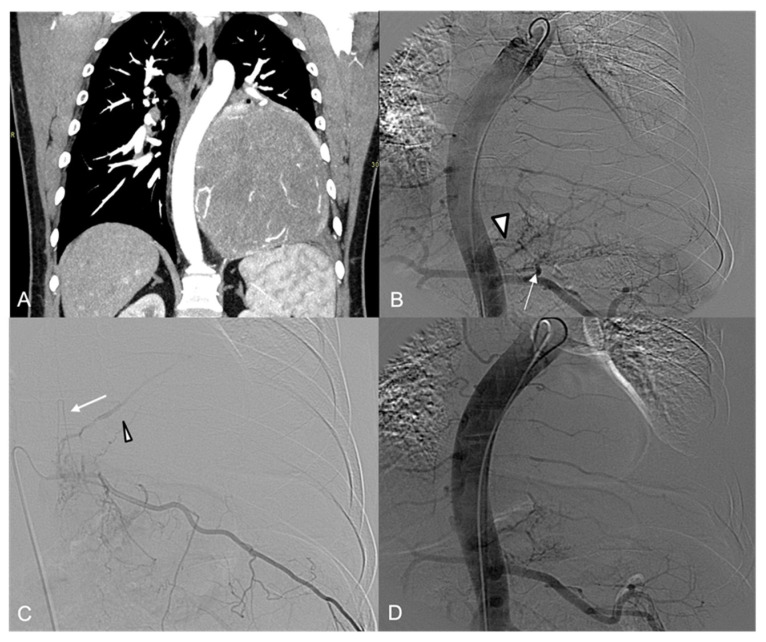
(A) CT Angiography, coronal view: large, highly vascular mass in the left hemithorax dislodging the thoracic aorta. (B) Preliminary aortography shows the rich vascularization of the mass, with branches arising mainly from the left phrenic artery (arrow) and the first left lumbar artery (arrowhead). (C) Selective catheterization of the X intercostal left artery shows the presence of the Adamkiewicz artery (arrow) and a minimal supply to the tumor (arrowhead). (D) Highly reduced tumor vascularization at the final aortography.
